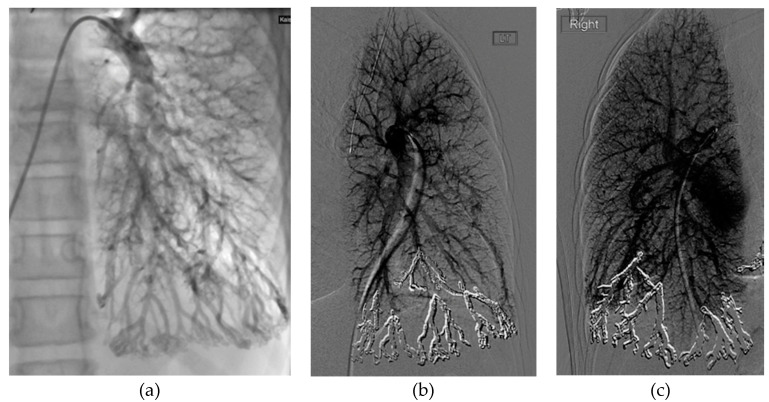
9-year-old female with genetically confirmed HHT, mild growth and developmental delay, presenting with dyspnea on exertion and chronic hypoxemia (baseline oxygen saturation of 60–80%) requiring supplemental oxygen. CT chest (not shown) showed diffuse PAVMs affecting all segments of both lungs. (a) Initial pulmonary angiogram of the left lung demonstrates diffuse PAVMs, most pronounced in the basal left lower lobe and lingula. (b) Final pulmonary angiogram of the left lung following peripheral-to-central embolization of the left lower lobe and lingula with implantation of 20 coils. (c) Final pulmonary angiogram of the contralateral right lung, performed 1 month after the left-sided embolization. Similar to the left lung, embolization was performed of the dominant PAVMs of the right lower lobe basilar segments. Despite the extensive embolization, the patient had no improvement in baseline oxygen saturation or functional status.
